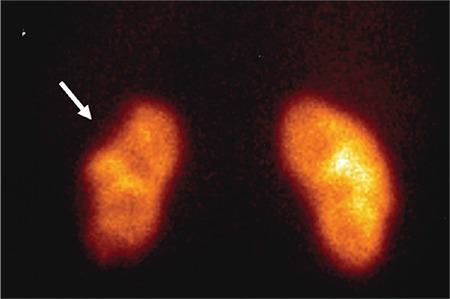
99mTc-dimercaptosuccinic acid renal scintigraphy showing cortical defects in the upper and lower lateral poles of the left kidney (anterior position).
